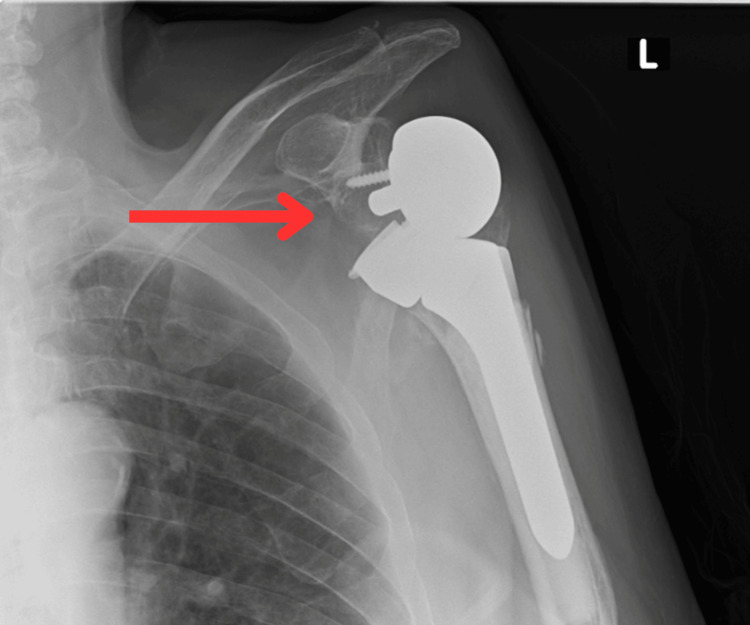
Post first open revision radiograph
